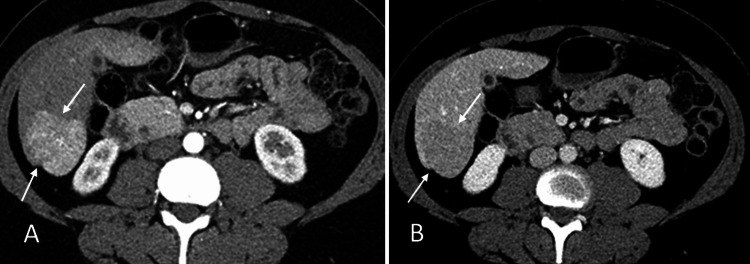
Contrast-enhanced CT abdomen and pelvis. Axial sections (A, B) demonstrate a well-defined enhancing lesion in the inferior right lobe of the liver with a central scar and washout of contrast on the delayed scan (white arrows in A and B) suggestive of focal nodular hyperplasia (FNH).
